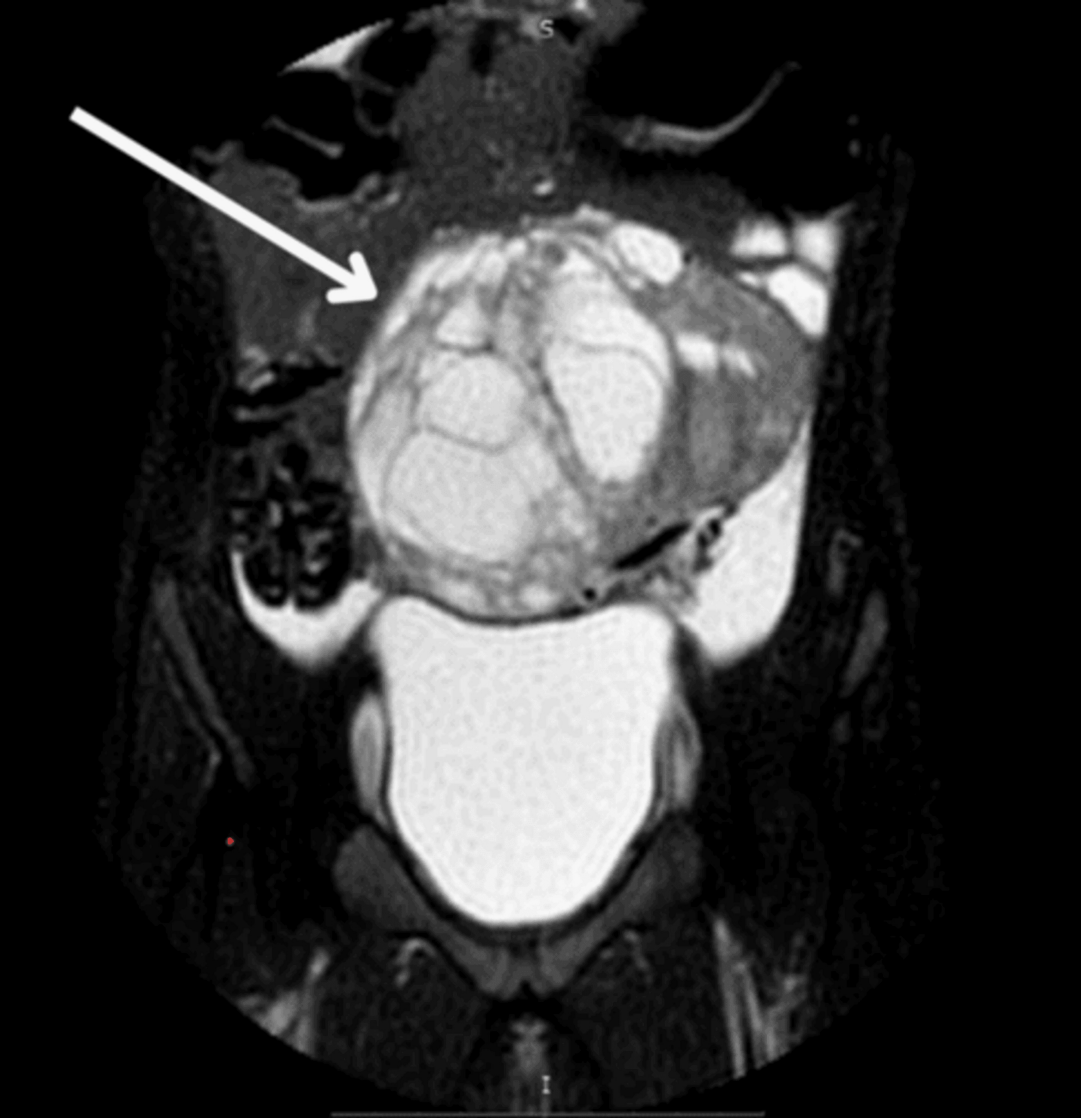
Coronal T2-weighted MRI image showing a multilocular pelvic mass (arrow) with hyperintense features The mass demonstrates its relationship to adjacent pelvic organs without evidence of invasion MRI: magnetic resonance imaging
